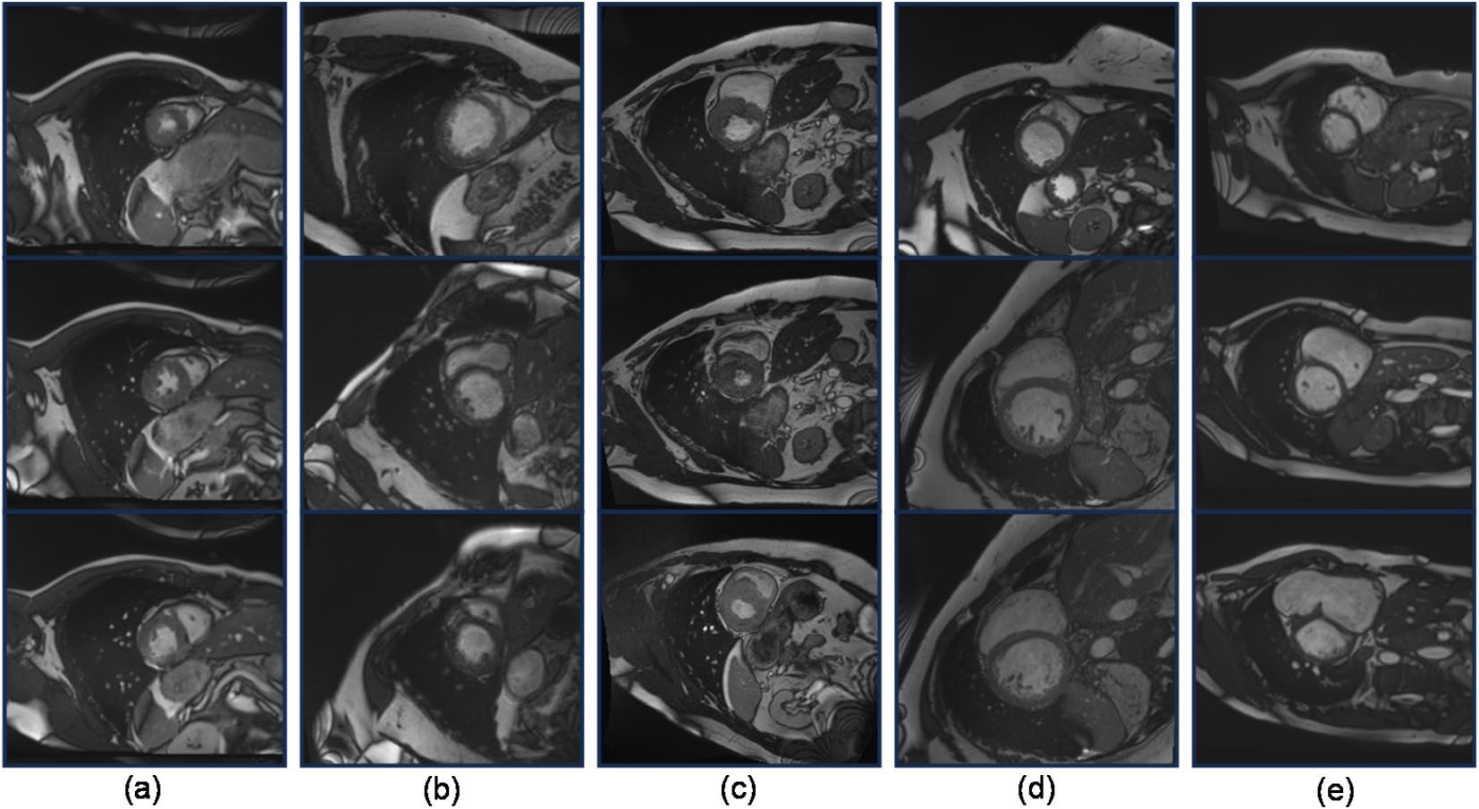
Cardiac MRI medical images in an ACDC dataset. (a) Normal Cardiacs; (b) Previous Myocardial Infarction; (c) Hypertrophic Cardiomyopathy; (d) Dilated Cardiomyopathy; (e) Abnormal Right Ventricle.
